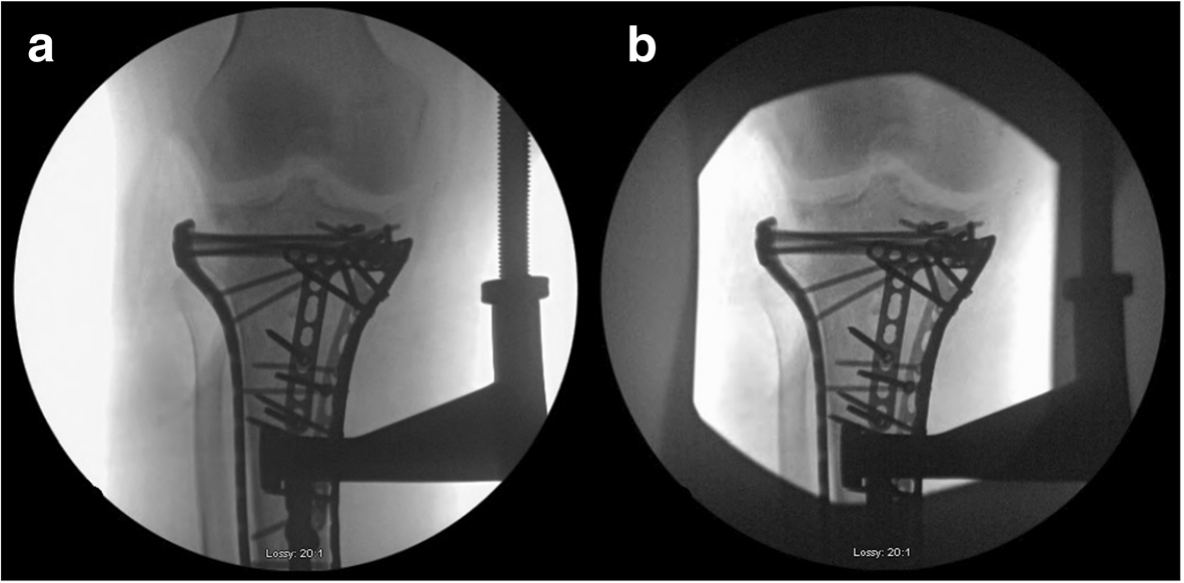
a shows a standard x-ray taken without collimation. b is an x-ray taken with collimation; collimation helps reduce exposure and may also help produce sharper images
